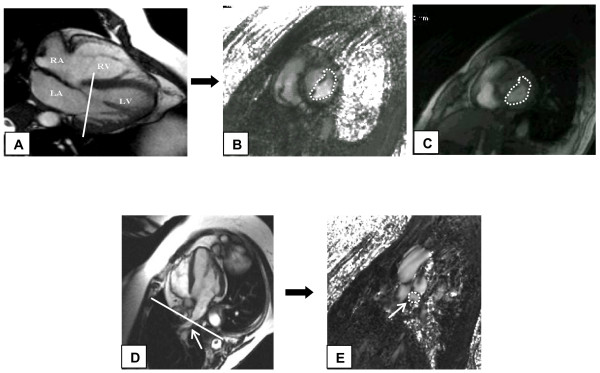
Image acquisition of velocity encoded (VENC) cine cardiovascular magnetic resonance imaging (CMR) at the level of mitral valve (MV). A) Cine 4 chamber image demonstrating the prescription of slice at the level of the tips of the MV leaflets. B) VENC CMR short axis image at the level of MV leaflet tips. Contour is drawn on the leaflets as shown including the whole cross-section of the mitral inflow. C) Magnitude image corresponding to the phase image. Note that the entire MV plane is interrogated as compared to the ice-pick view of conventional transthoracic echocardiography (TTE) imaging. D) Cine 4 chamber image demonstrating the position of slice 1 cm inside the right superior pulmonary vein (arrow) entrance into the left atrium. E) VENC CMR cross-section image of the right superior pulmonary vein with contours (arrow).
